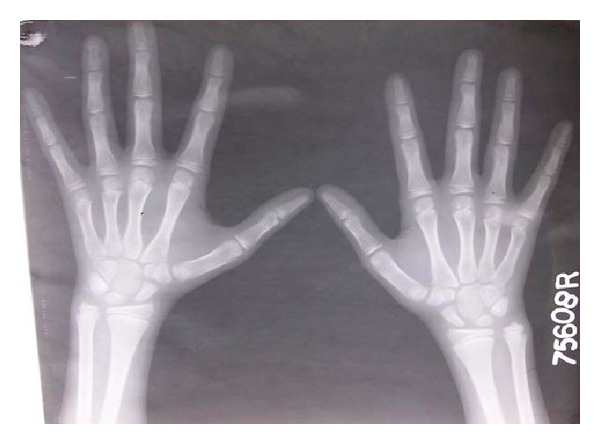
Hand-wrist radiograph for growth-spurt assessment.
